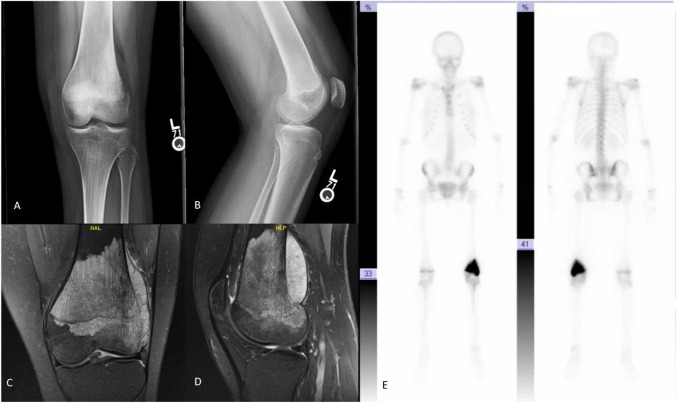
A, AP radiograph of the patient's left knee. B, Lateral radiograph of the patient's left knee. C, Coronal MRI cuts of the patient's left knee. D, Sagittal MRI cuts of the patient's left knee. E, Whole body bone scan of the patient with increased uptake in the left knee.
